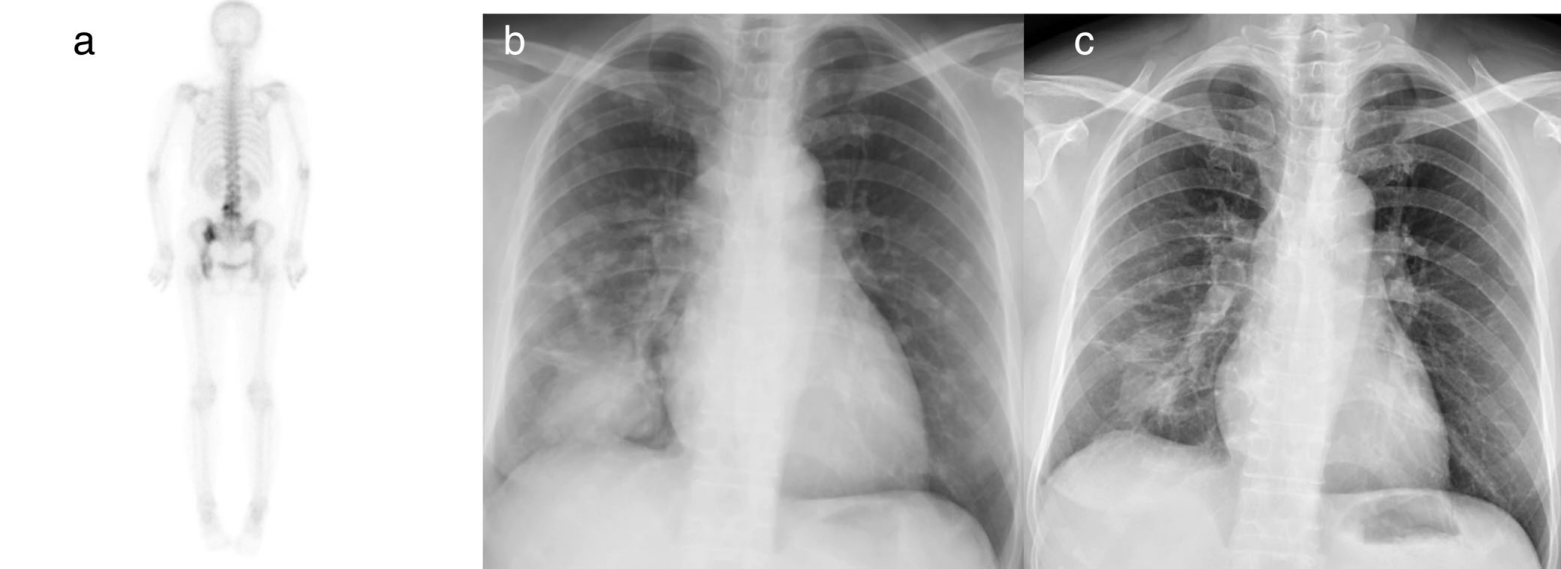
Findings of bone scan and chest radiography performed before and after administration of combination therapy. (a) Bone scan findings showed metastases at the lumbar spine. (b) A huge mass in the right lower lung (RLL) with lung‐to‐lung metastasis was observed on chest radiograph. (c) Chest radiograph revealed the RLL mass and bilateral lung nodules had significantly decreased in size
